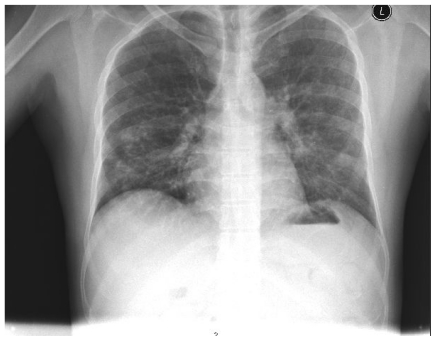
Chest X-ray showing marked improvement with steroid treatment
